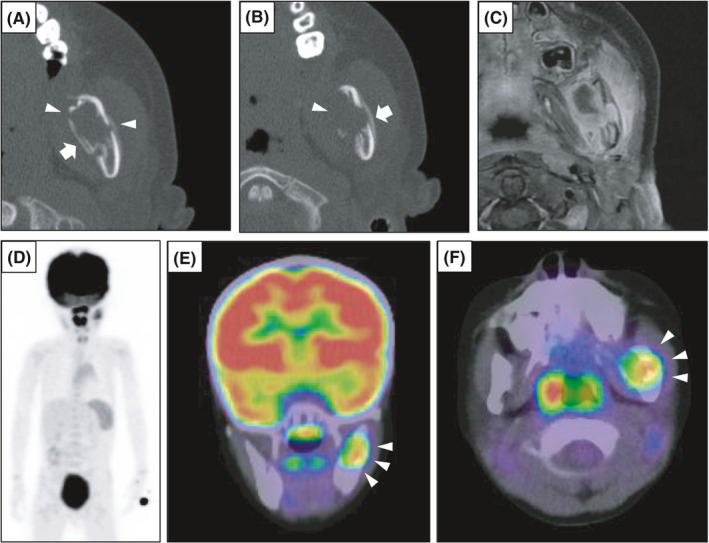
Radiological features. A, B, CT scan showing osteolysis with indistinct borders in the left mandible. A rupture (arrowhead) and periosteal reaction (arrow) was found in the buccolingual cortical bone. C, Contrast‐enhanced MRI T1‐weighted imaging showing a mass extending outside the buccolingual bone. D‐F, Bone scintigraphy and PET‐CT showing abnormal accumulation only in the left mandible (arrowhead)
